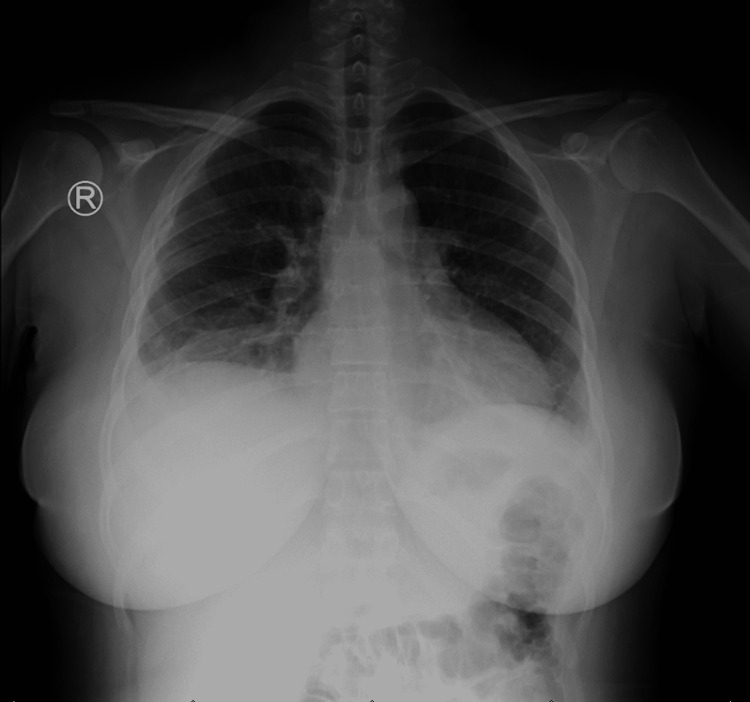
Chest x-ray posteroanterior (PA) view shows a hazy left costophrenic angle with sub-pulmonic effusions on the right side.
